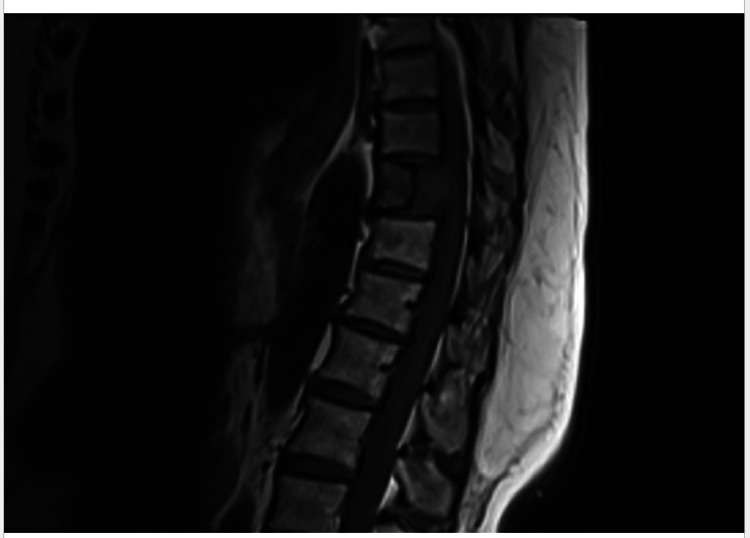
MRI showing a lytic lesion at T10.
